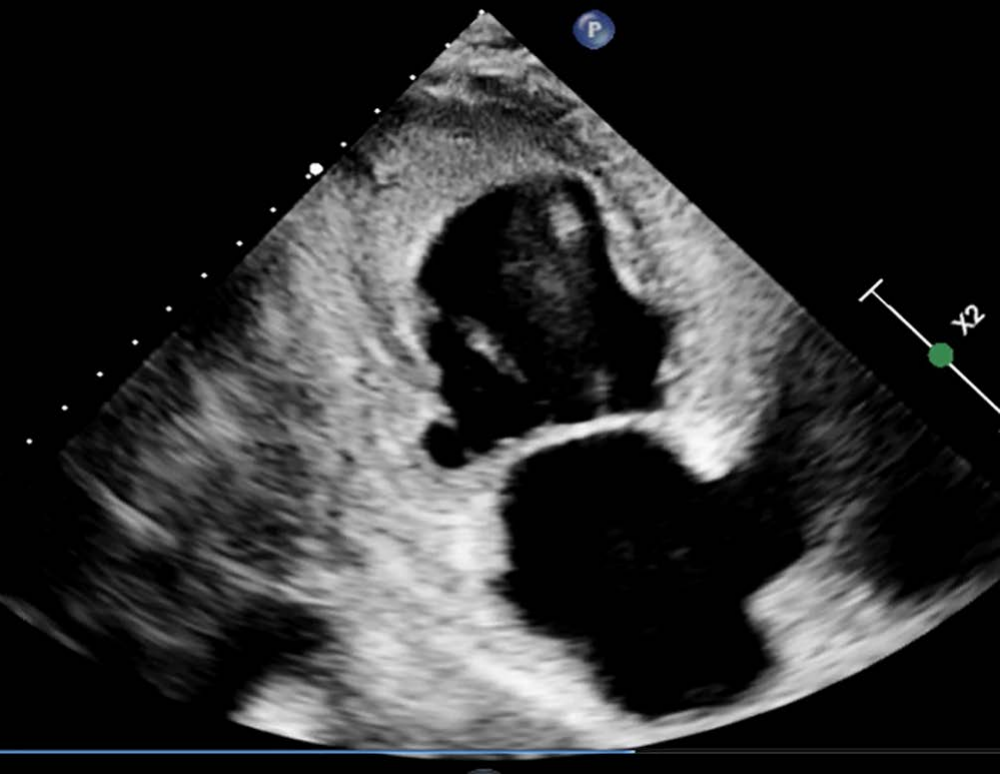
Echocardiogram showing left ventricular wall thickening with left atrial and ventricular enlargement.
